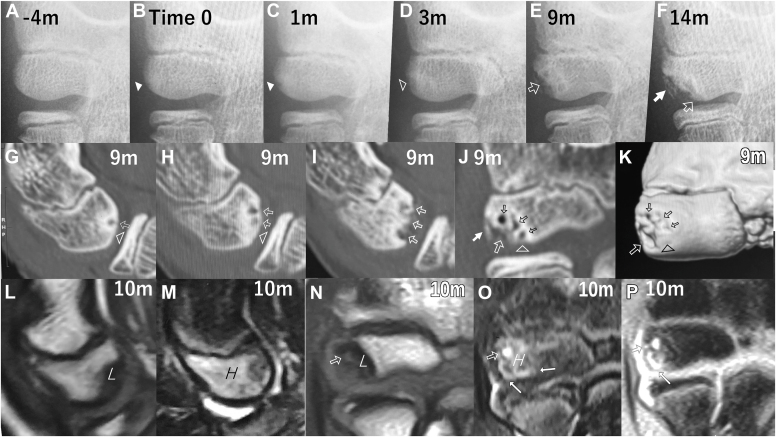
Case 1, a 10.5-year-old boy with osteochondritis dissecans of the capitellum. (A-F) Anteroposterior radiographs of the elbow at 45° of flexion (APR45). (A) APR45 at initial presentation showing no abnormal findings of the capitellum. (B) APR45, taken 4 months after initial presentation, showing the first detectable feature that was extremely subtle rarefaction surrounded by sclerosis within a limited area of the ossifying subchondral bone surface in the lateral aspect of the capitellum (*arrowhead*). This time was assumed to be Time 0. (C, D) APR45, taken 1 and 3 months after Time 0, showing progression from the rarefaction (*arrowhead*) to flattening (*open arrowhead*). (E) APR45, taken 9 months after Time 0, showing subchondral bone depression (*open arrow*) and an uneven bony floor surrounded by osteosclerosis. He was diagnosed to have OCD at radiographic stage I and was treated nonoperatively with activity restriction. (F) APR45, taken 14 months after Time 0, showing subchondral depression expanding to the central aspect of the capitellum (*open arrow*) and endochondral ossification (*white arrow*) along the original capitellar bone surface in the lateral. (G-K) CT images taken 9 months after Time 0. (L-P) MRI taken 10 months after Time 0. (G, H, I) Sagittal CT images, reconstructed along a plane with 10° in valgus,^41^ showing flattening (*open arrowheads*), small subchondral bone cysts (G, H; *open arrows*), and depression (I, *open arrows*) surrounded by osteosclerosis. (J, K) Coronal CT image, reconstructed along a plane with a tilting angle of 55°,^41^ and 3D-CT image showing flattening (*open arrowhead*), a partial bone defect (*large open arrow*), small cyst-like depressions (*small open arrows*) surrounded by osteosclerosis, and ossification in the lateral (J, *white arrow*). (L, M) Sagittal MRI taken along a plane with 10° in valgus.^41^ (N, O, P) Coronal MRI taken along a plane with a tilting angle of 55°.^41^ (L, N) T1-weighted MRI showing subchondral bone cyst (*arrow*) and low signal area (*L*) consistent with osteogenic changes and bone defect. (M, O, P) Fat-suppressed T2-and T2∗-weighted MRI showing a high-signal interface (*white arrow*), a subchondral bone cyst (*open arrow*), and high signal area (*H*) consistent with bone marrow edematous changes. *3D*, three dimensional; *CT*, computed tomography.
